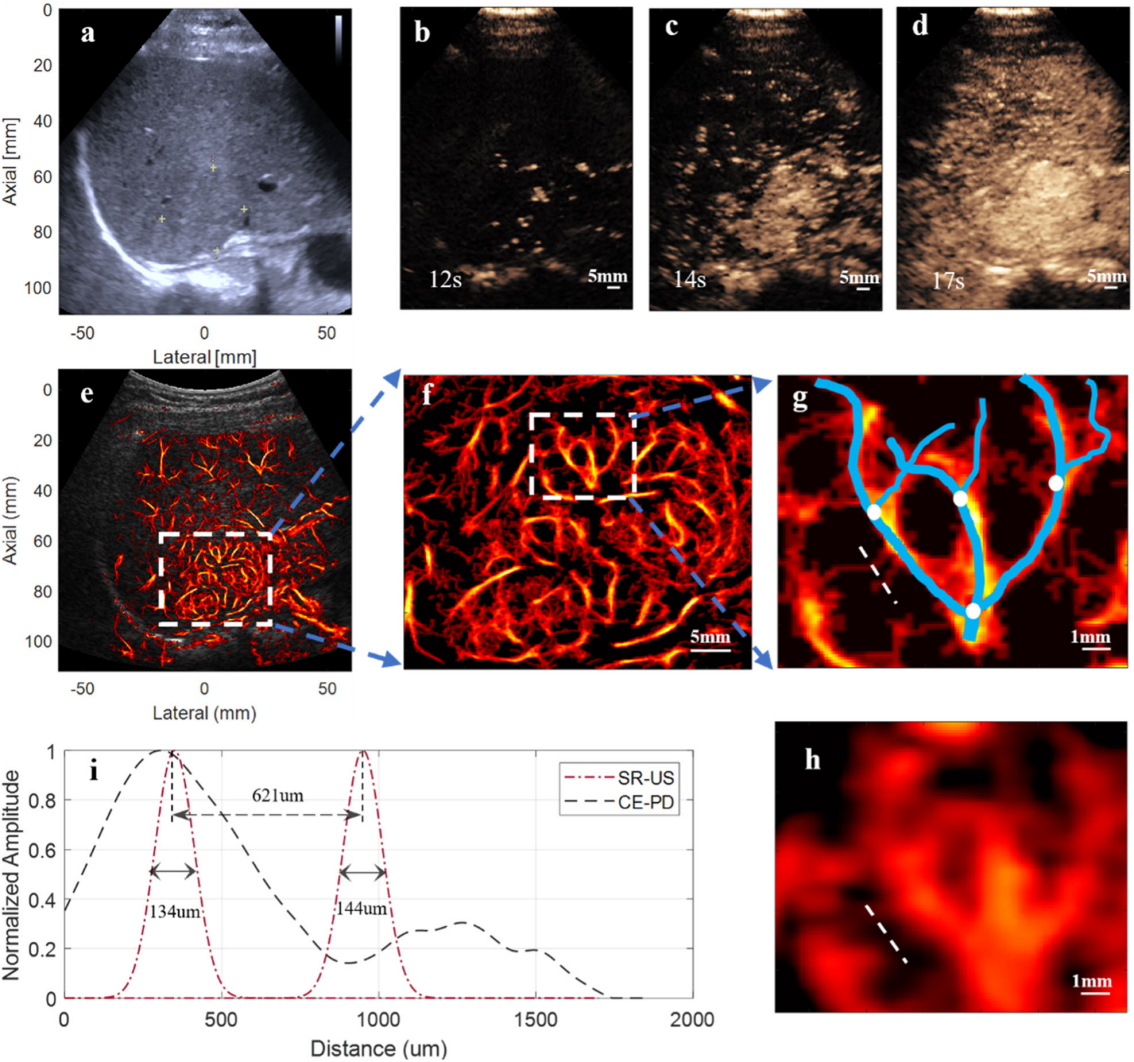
Focal nodular hyperplasia in a 38-year-old female patient. a B-mode image. b–d Contrast-enhanced ultrasound image. e Super-resolution ultrasound (SR-US) microvascular density image overlaid on the ultrasound B-mode image. f The lesion was zoomed-in region as indicated by the white rectangle in e. g Magnified region indicated by the white rectangle in f. h Contrast-enhanced power doppler (CE-PD) image of the same region in g. i Vessel cross-section intensity profile of SR-US and CE-PD image. Intensity profile of the vessel cross-section, delineated by the white dashed line in the SR-US image (g) and the CE-PD image (h), is represented by the red and black dashed curves, respectively
